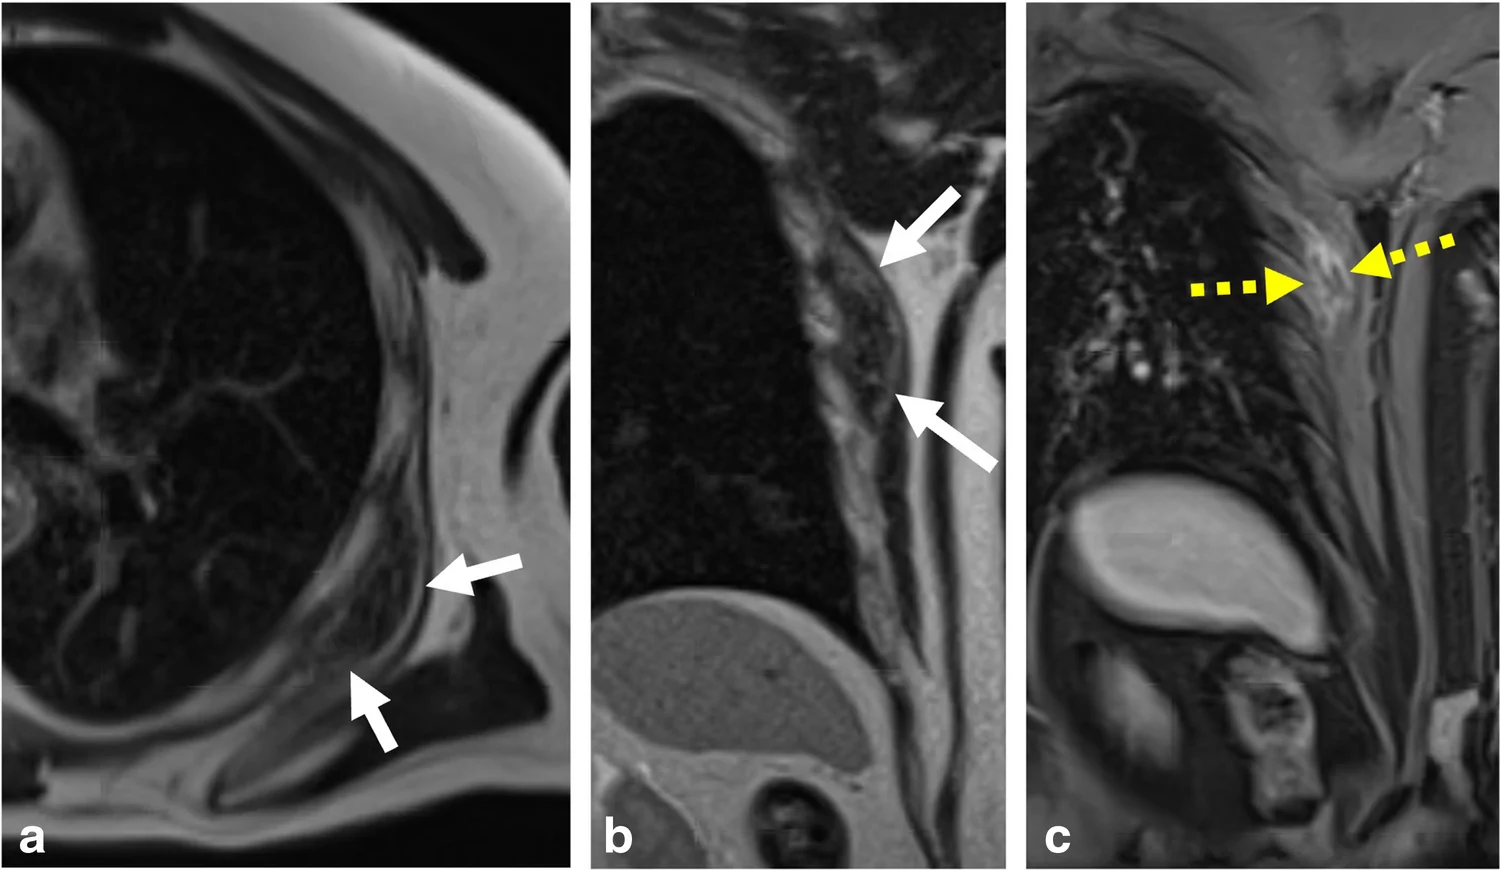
Three-month follow-up MRI after percutaneous cryoablation of elastofibroma dorsi. a Axial (TE = 98 ms; TR = 1400 ms) and b coronal (TE = 100 ms; TR = 1400 ms) HASTE T2-weighted MR images demonstrate interval size reduction of the treated lesion (white arrows). c Post-contrast coronal fat-suppressed 3D T1-weighted image (VIBE, TE = 2.39 ms; TR = 7.02 ms) shows internal non-enhancing necrotic change consistent with post-ablation effect (dotted yellow arrows)
